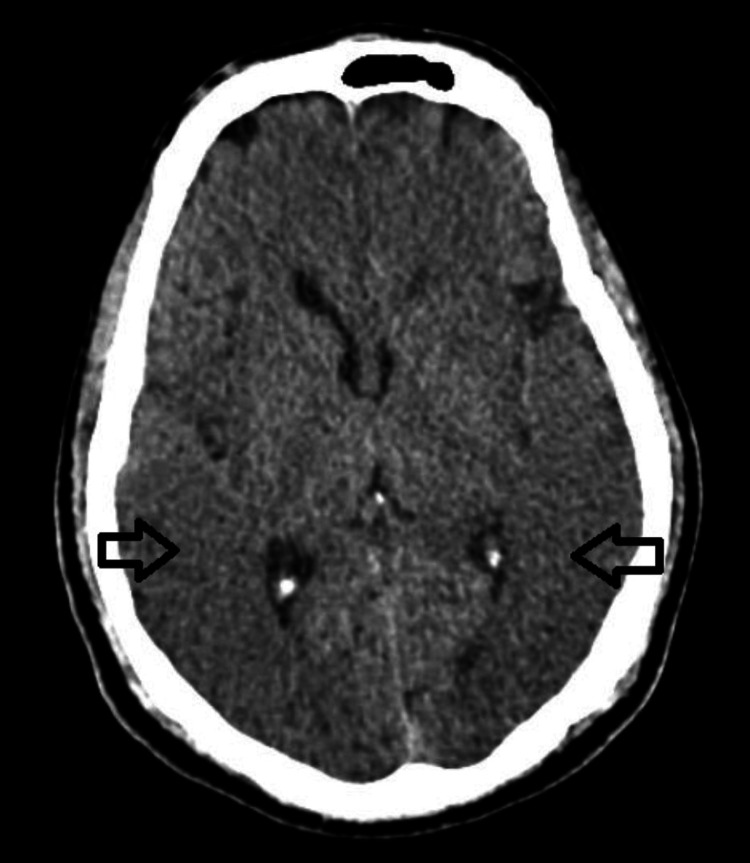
Non-contrast computerized tomography (CT) brain scan; arrows indicate the loss of gray-white matter differentiation with effacement of sulcal gyral spaces in the bilateral temporal and parietal occipital lobe
